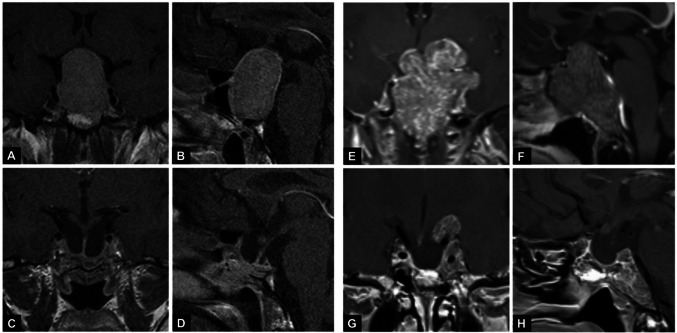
T1-weighted MRI in coronal (A, C, E, G) and sagittal (B, D, F, H) planes of a 39-year-old patient before (A, B) and after (C, D) a single transsphenoidal operation with GTR and a 48-year-old patient before (E, F) and after (G, H) a combined approach (TS + TK) with incomplete tumor resection. Both patients suffered from non-functioning pituitary adenomas and presented with visual compromise, which recovered completely after surgery
